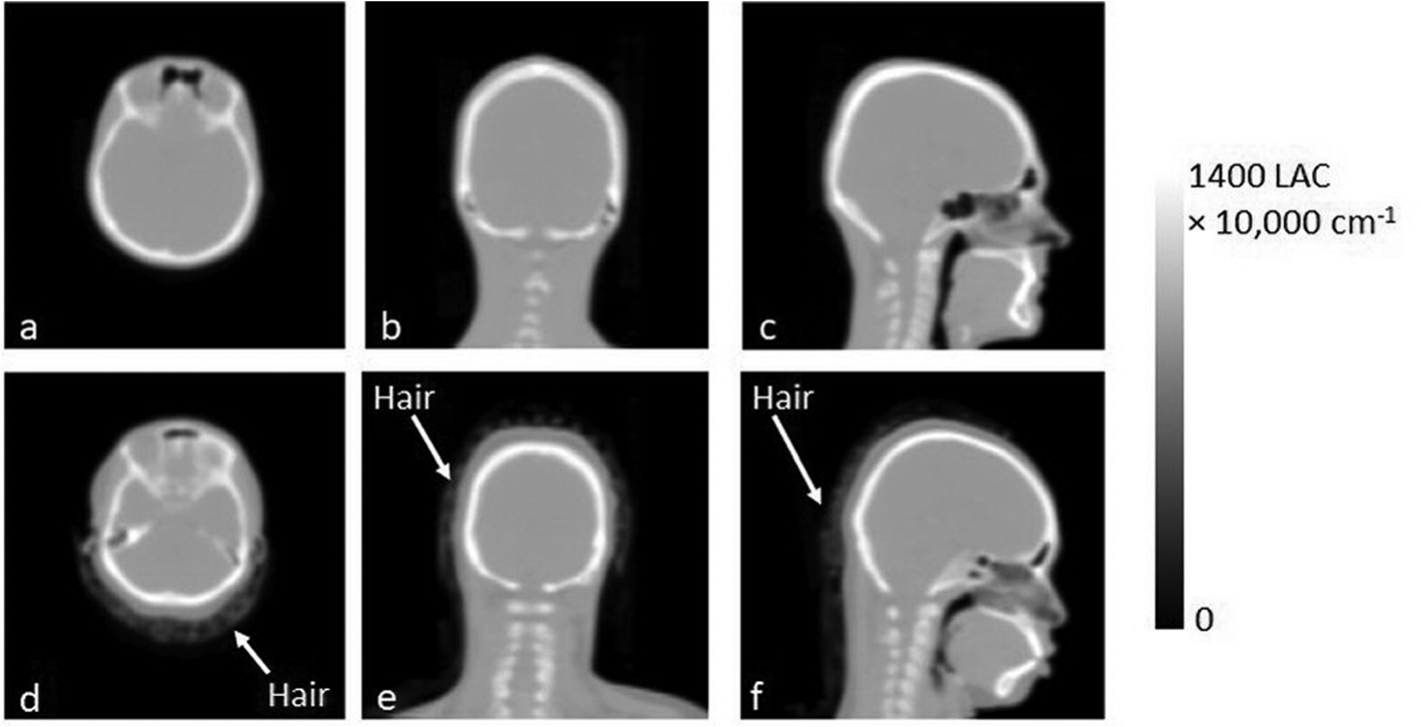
a–c CT-derived μ-map of a patient who has short/low density hair. d–f CT-derived μ-map of a patient with dense hair surrounding the head (arrows indicate location of the hair in each image)
